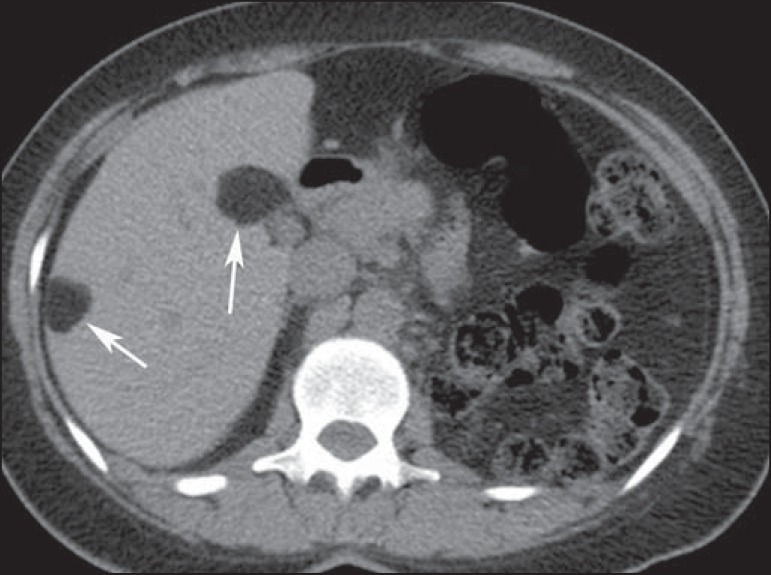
A 27-year-old man with tuberous sclerosis complex. Axial unenhanced CT
